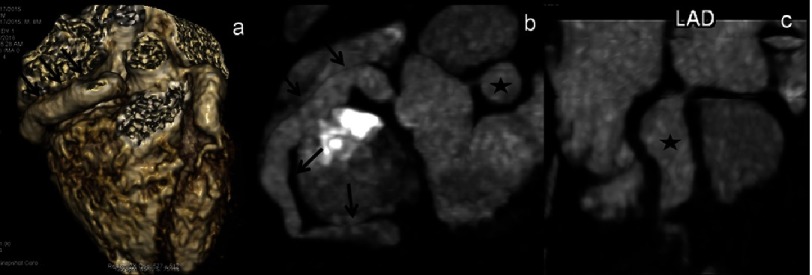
(a–c): CTCA VRT (a) and curved (MIP) images (b & c) of an 8 year male child in acute phase show aneurysmal dilation of entire RCA (a & b - thin arrows) and a giant aneurysm in proximal LAD (b & c - asterisk). 2D-transthoracic echocardiography detected aneurysm in both the arteries; however it failed to delineate the extent of involvement of RCA.
